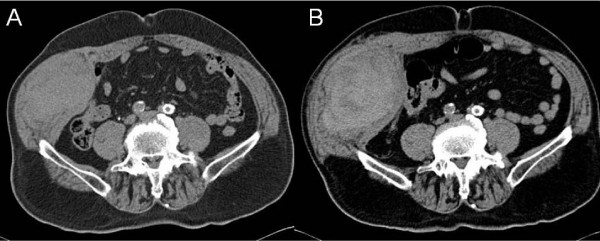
(A) Abdominal computed tomography (CT) shows the extensive hematoma in the right lateral abdominal wall 2 hours after carotid artery stenting. (B) Abdominal CT clearly shows enlargement of the hematoma 7 hours after the first CT.
